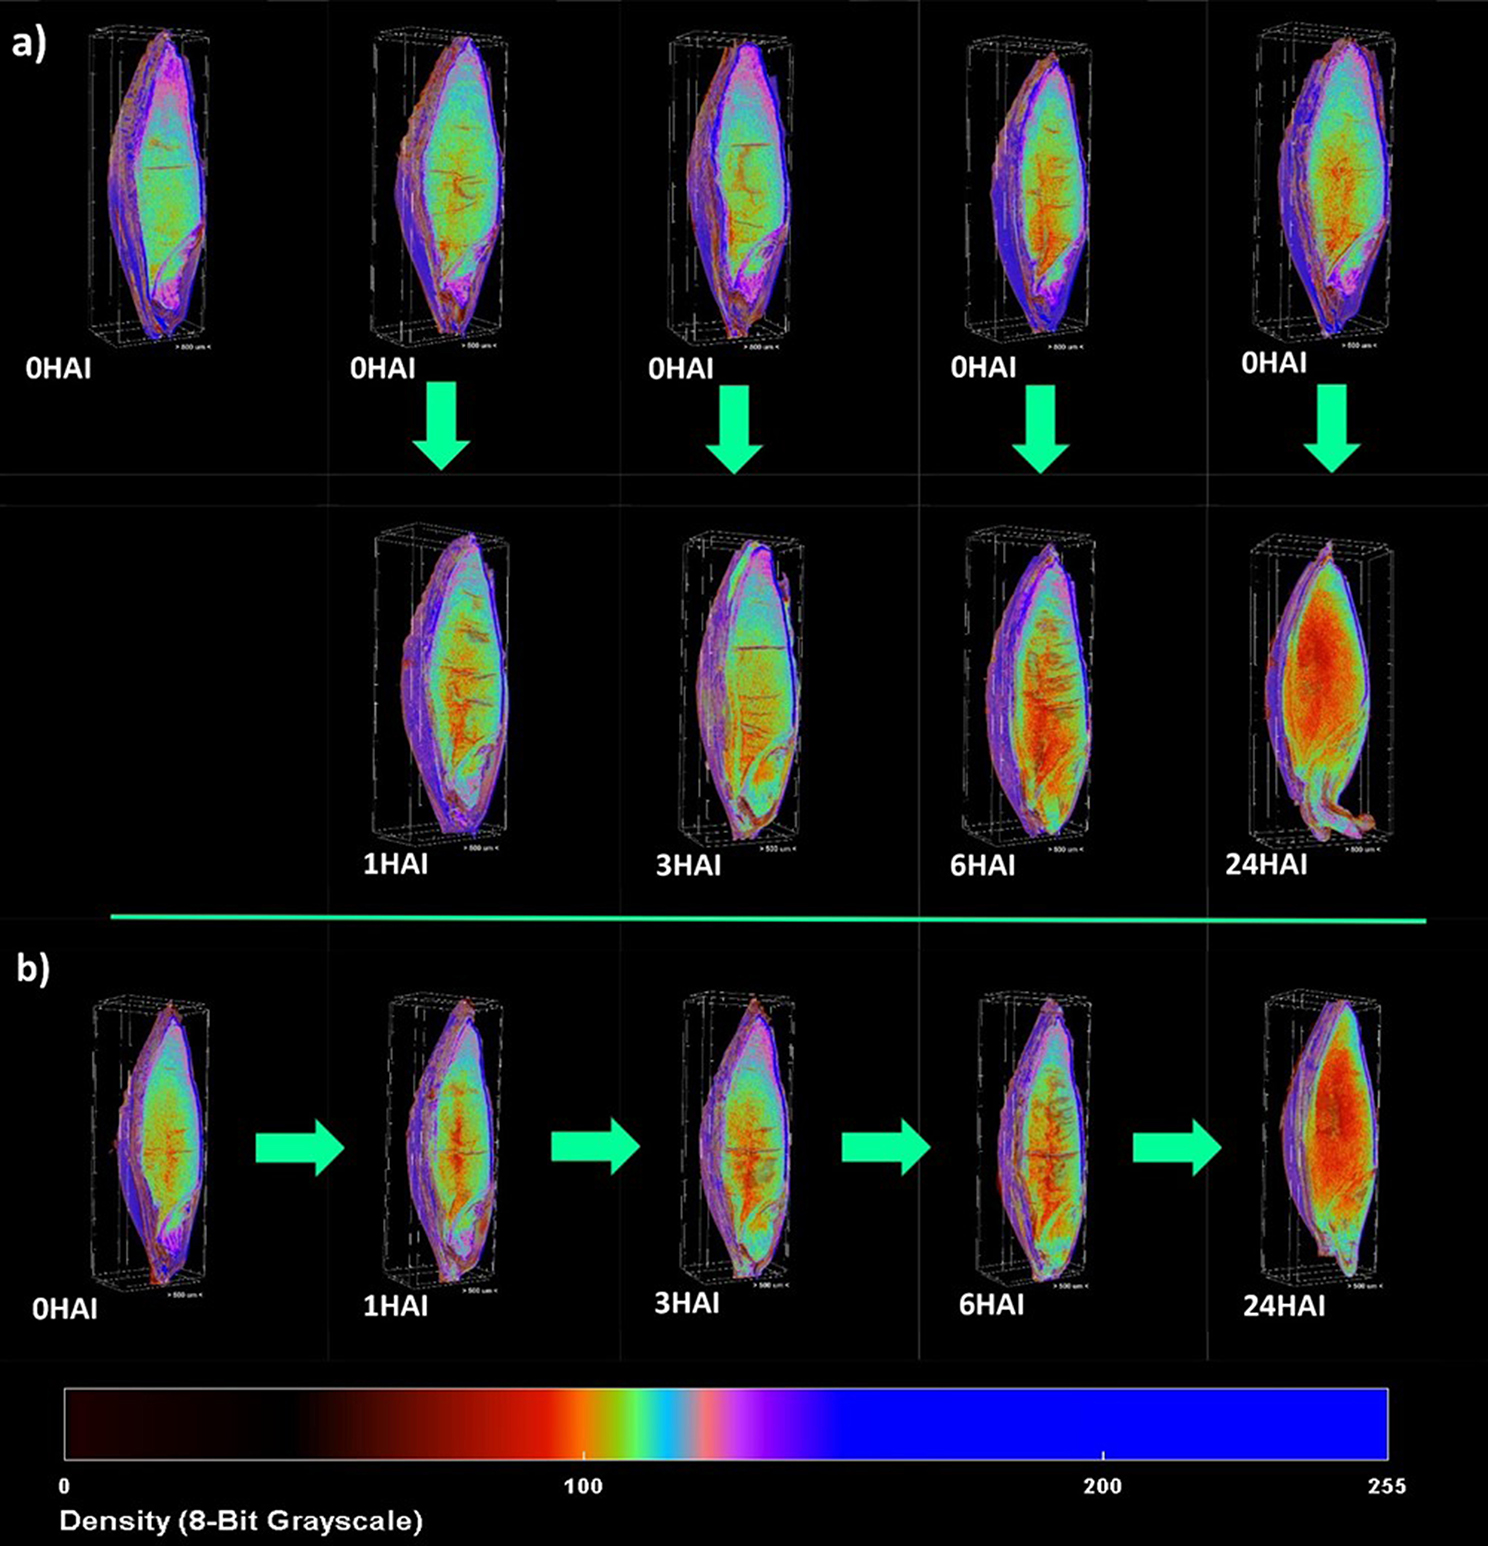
µ-CT images of (a) double scan grains which were scanned at 0 HAI, then at set timepoints and (b) a singular grain which was repeatedly scanned at each set timepoint. The pseudo coloring represents comparative density
